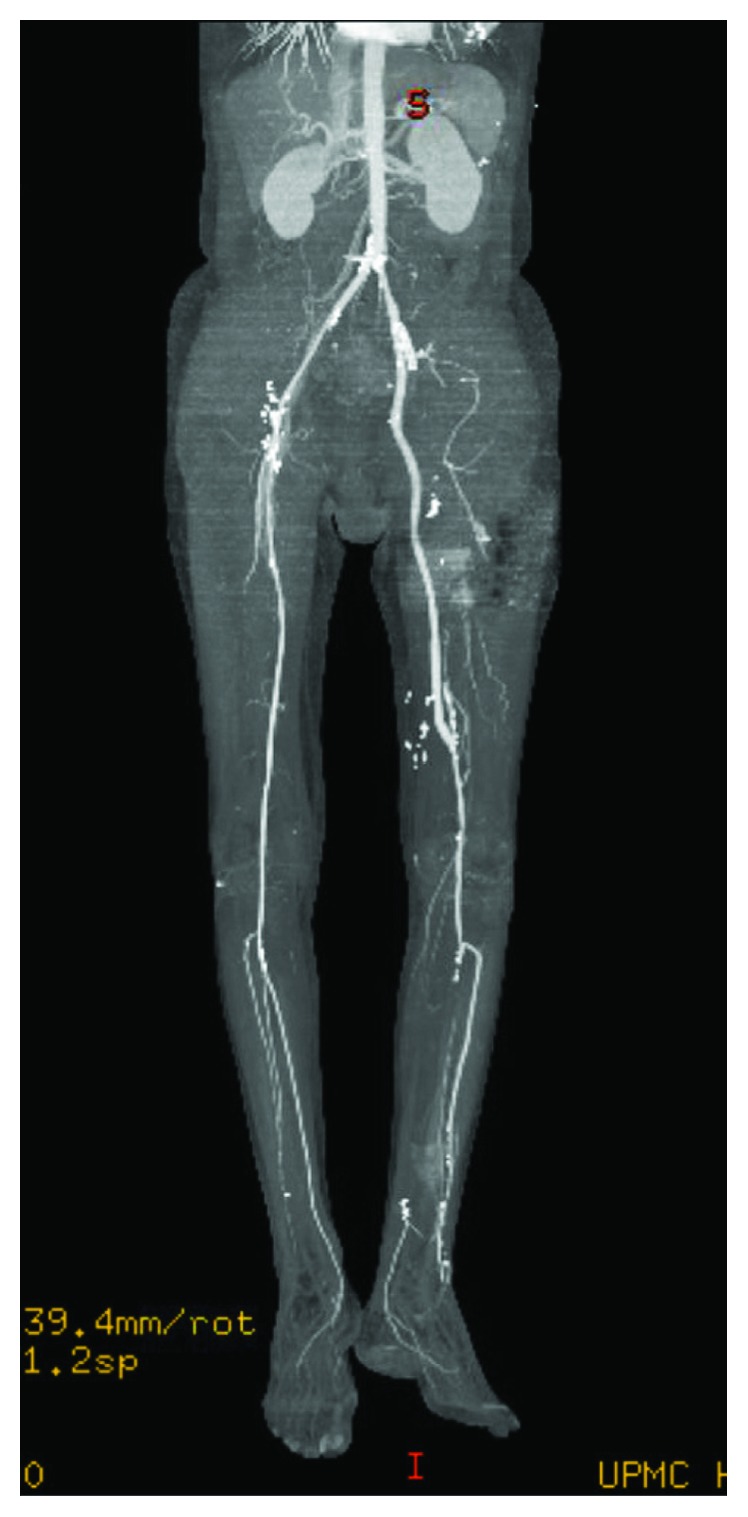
Postoperative CT angiogram of obturator bypass.
